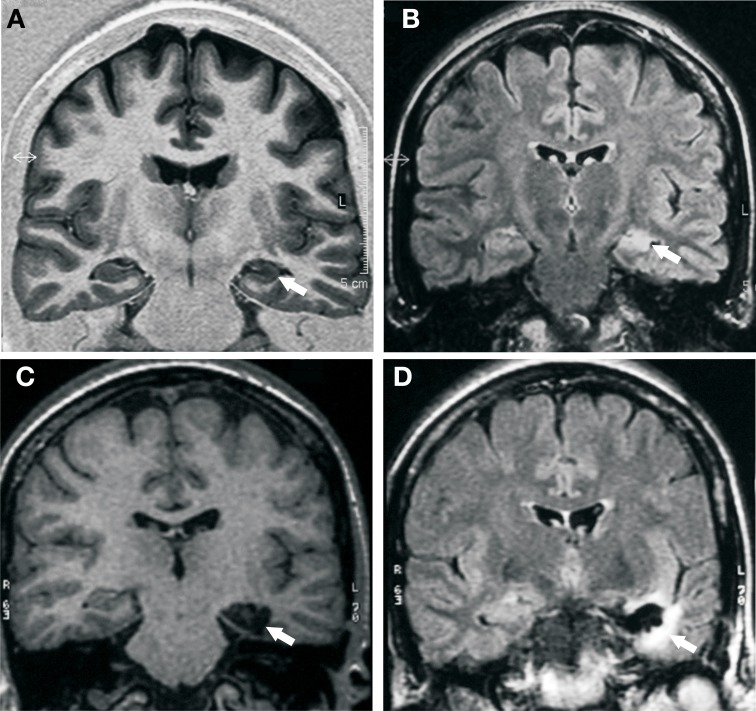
Pre- and post-surgical MRI study. (A) Pre-surgical MRI; IR coronal image shows reduction of left hippocampal size, with hypointensity, and loss of internal differentiation (white arrow). (B) Pre-surgical MRI; Flair coronal image reveals hyperintensity and decreased hippocampal size (white arrow). (C) Post-surgical MRI; T1 coronal image shows the absence of the hippocampal gyrus, the subiculum, and the parahippocampal gyrus, medial to collateral sulcus (white arrow). (D) Post-surgical MRI; Flair coronal image hyperintensity is observed, surrounding the surgical cavity, secondary to gliosis (white arrow).
